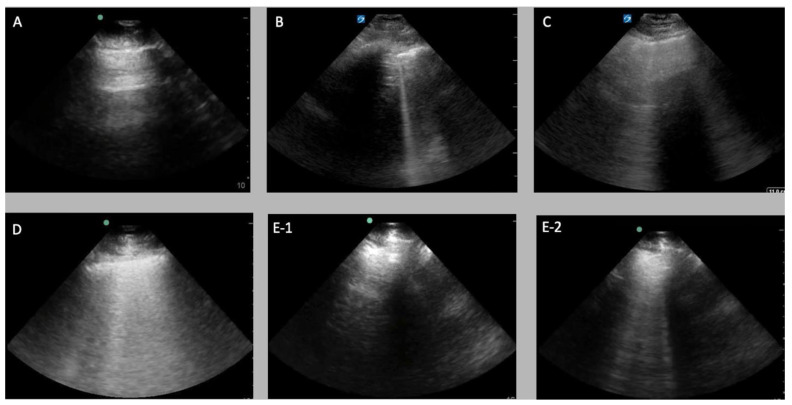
Still image representations of the various lung ultrasound artifacts, severity and heterogeneity applied as labels to our data: (A) A-line pattern (normal lung) class; (B) fewer than 3 B lines class; (C) moderate B line class; (D) severe B line class; (E) 2 frames showing heterogeneity within the same clip with A lines (E-1) followed by moderate B lines (E-2) sliding in to view.
